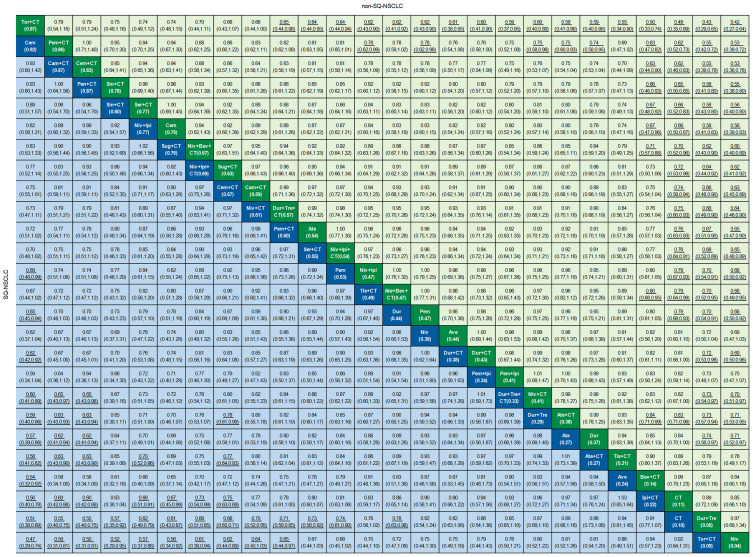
Pooled OS estimates of multiple comparisons in network meta-analysis. Treatment regimens are presented in order of OS ranking from high to low according to SUCRAs. Dark blue represents the regimens for SQ-NSCLC (SUCRA values), and dark green represents the regimens for non-SQ-NSCLC (SUCRA values). Data are HRs (95% CrIs) for column-defined treatment versus row-defined treatment for SQ-NSCLC (lower triangle) and row-defined treatment versus column-defined treatment for non-SQ-NSCLC (upper triangle). Significant results are underlined.OS, overall survival; SUCRA, surface under the cumulative ranking; HR, hazard ratio; CI, confidence interval; SQ-NSCLC, squamous non-small cell lung cancer; Anl, anlotinib; Ate, atezolizumab; Ave, avelumab; Ben, benmelstobart; Bev, bevacizumab; Cam, camrelizumab; Cem, cemiplimab; Dur, durvalumab; Ipi, ipilimumab; Ivo, ivonescimab; Niv, nivolumab; Pem, pembrolizumab; Pen, penpulimab; Ser, serplulimab; Sin, sintilimab; Sug, sugemalimab; Tis, tislelizumab; Tor, toripalimab; Tre, tremelimumab; CT, chemotherapy.
